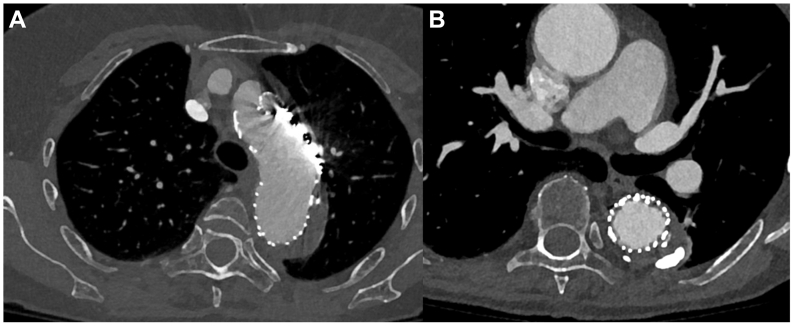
(A) Computed tomography angiography (CTA) 2 months after percutaneous transthoracic retrograde coil embolization of a gutter endoleak demonstrating complete resolution of the endoleak and (B) the presence of Histoacryl glue at the level of the puncture site.
